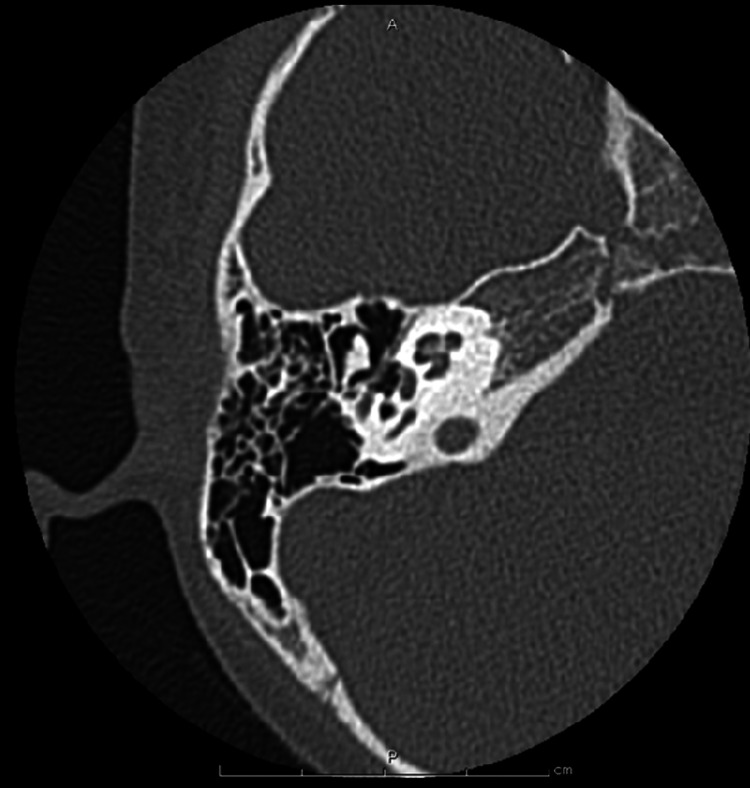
Imaging of Case 2: temporal bone CT (right) Healthy side. CT, computed tomography.
